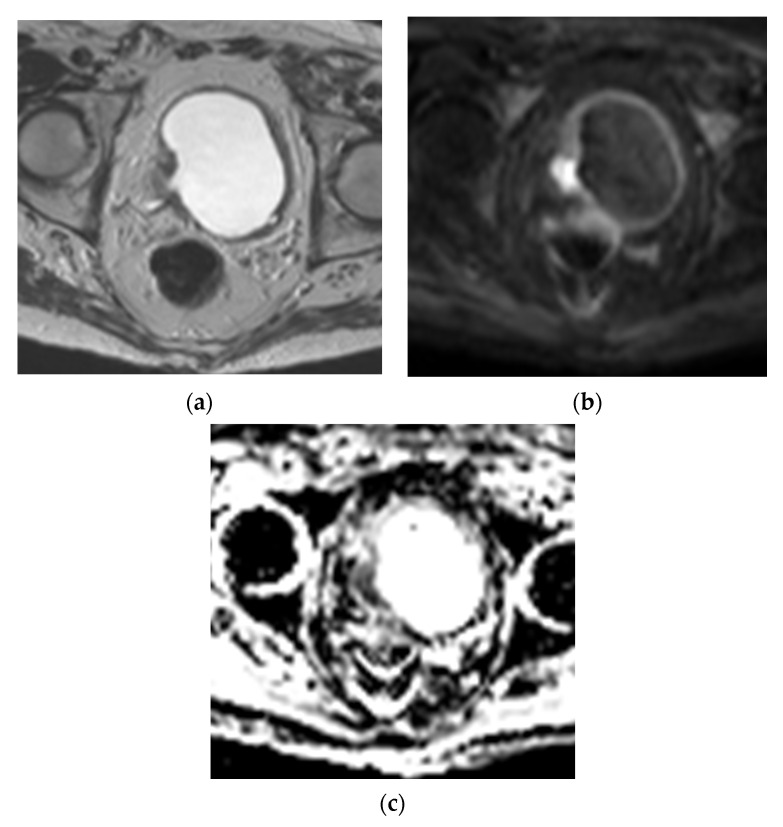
Muscle invasive bladder cancer on MRI (a) Axial T2WI shows a muscle invasive mass in the right bladder wall, seen as a T2 intermediate signal lesion (solid white arrow) invasive into the muscularis propria which is T2 hypointense. The mass is located at the ureterovesicular junction, causing hydroureter (dashed white arrow). (b) Axial DWI image, showing the hyperintense mass invading the muscularis propria, which is T2 intermediate signal on DWI. (c) ADC map showing hypointensity in the area of DWI hyperintensity, confirming restricted diffusion.
